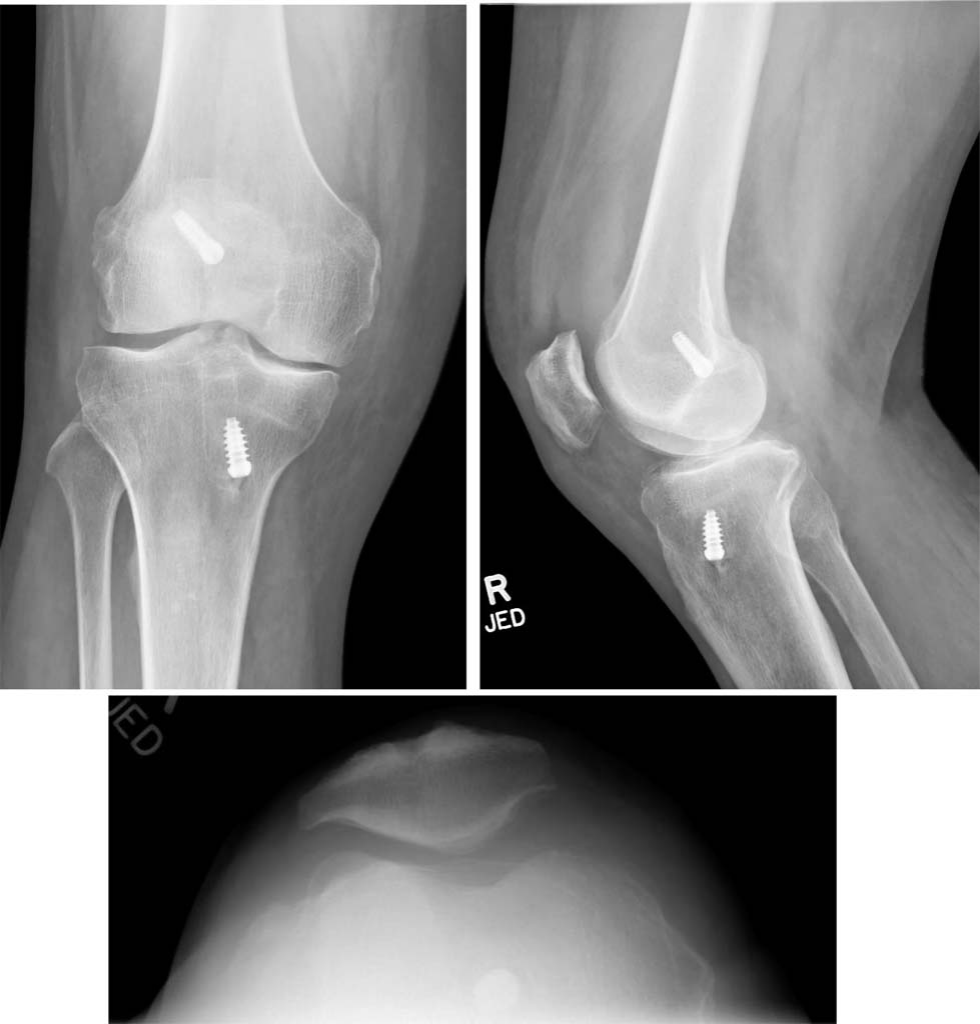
Radiograph showing standard Anterior-Posterior (AP), lateral and sunrise patella views of the knee showing earlier ACL reconstruction, moderate-to-severe tricompartmental osteoarthritis, and moderate joint effusion.
